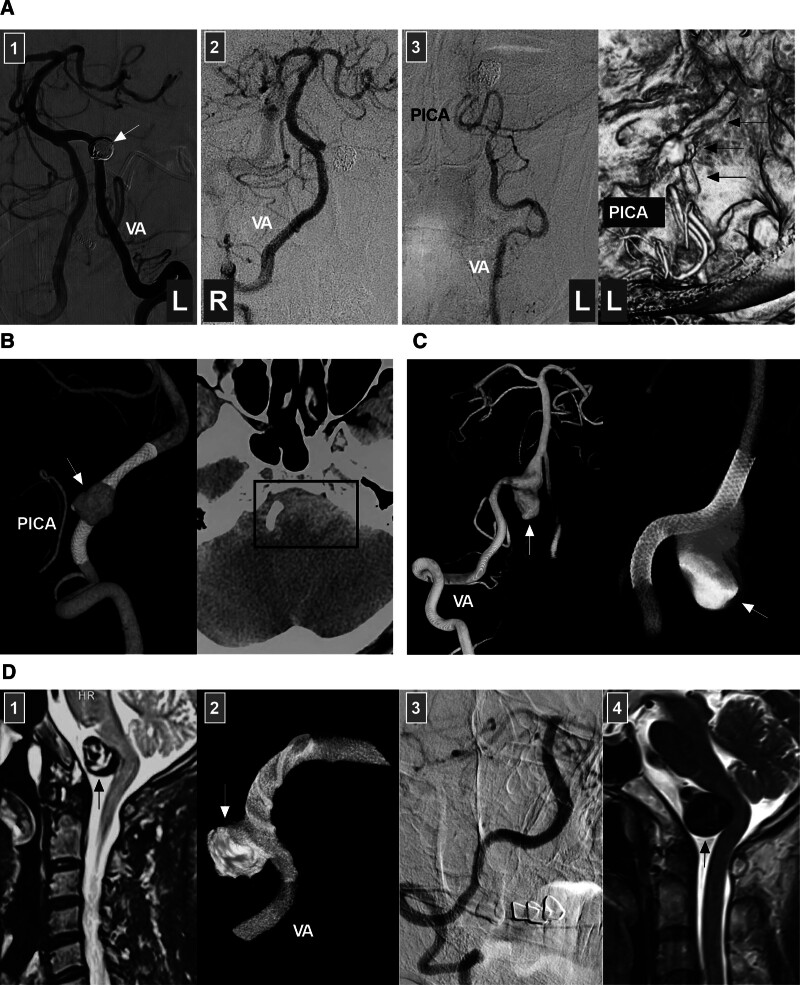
Complications of FD deployment. (A) VA occlusion after FD deployment: panel 1: DSA image of the left VA showing a coiled VA aneurysm (arrow) covered by an FD; panel 2: 6-month follow-up DSA image of the right VA showing that the vessel supplies the vertebrobasilar arteries; panel 3: DSA image of the left VA showing that the vessel terminates at the PICA and that the VA distal to the PICA is occluded (left panel); Reconstructive DSA image showing the FD device (arrows) and coils in the occluded left VA (right panel). (B) Hemorrhagic complications: left panel: vaso-reconstructive DSA image showing a VA dissecting aneurysm (arrow) treated with FD deployment; right panel: 6 h after EVT, computed tomography imaging shows subarachnoid hemorrhage (frame), indicating aneurysm rupture. (C) VA aneurysm with a mass effect after FD deployment: left panel: DSA image showing a large intracranial VA aneurysm (arrow); right panel: vaso-reconstructive DSA image showing FD coverage of the aneurysm (arrow). Postoperatively, the patient experienced weakness in the left upper and lower limbs, while MRI showed no hemorrhage or infarction. Three days later, the patient recovered, indicating that the aneurysm had expanded after FD deployment. (D) VA aneurysm with mass effect after FD deployment: panel 1: MRI showing a lesion (arrow) with a mass effect to the brainstem; panel 2: vaso-reconstructive DSA image showing the aneurysm (arrow) covered by the FD. panel 3: 6-month follow-up DSA image showing complete aneurysm occlusion; panel 4: follow-up MRI showing that the aneurysm (arrow) has not shrunk. DSA = digital subtraction angiography, MRI = magnetic resonance image, PICA = posterior inferior cerebellar artery, VA = vertebral artery.
